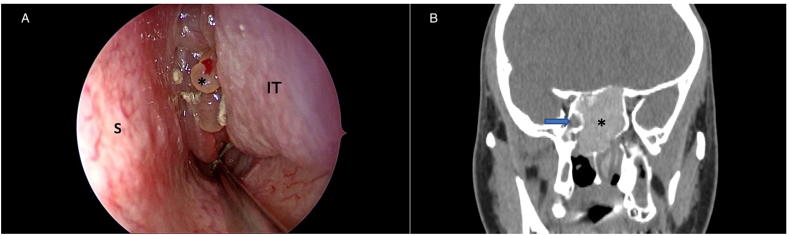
A) Polypoid mass *(asterisk)* in the left nasal cavity before surgery; S = nasal septum, IT = inferior turbinate; B) CT scan of the paranasal sinuses shows a hyperdense lesion *(asterisk)* in the left sphenoid sinus. The lesion expands the left sphenoid sinus cavity, making the size of the right sphenoid sinus cavity (*arrow*) extremely small.
